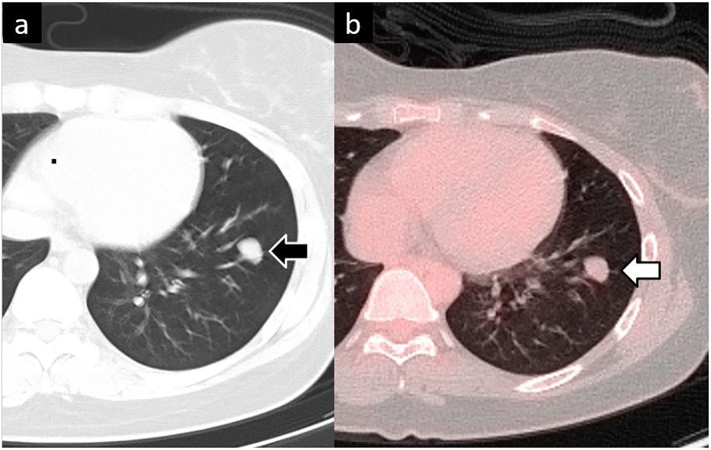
a) A chest radiograph showing the nodule noted on CT. The black arrow indicates the tumour. b) Chest radiograph of the nodule after 18 months of follow-up. The white arrow indicates the tumour.
